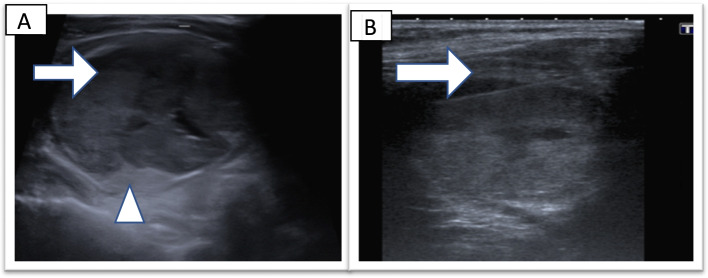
Thyroid ultrasound for patient 5; a 66 year old female with an incidental thyroid nodule identified while undergoing a carotid doppler ultrasound examination as part of a workup for transient ischaemic attack. Thyroid ultrasonography showing a 3.5 cm heterogeneous nodule with hypoechoic (arrow) and hyperechoic components (arrowhead) U3 (2A). Thyroid ultrasonography performed at the time of FNAB showing needle track advanced into the nodule (arrow) (2B)
